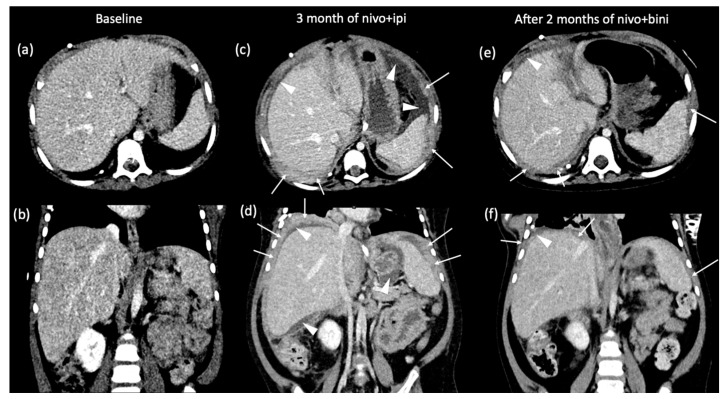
Axial (a) and coronal (b) contrast-enhanced CT of the abdomen at baseline are normal except for hepatomegaly. Axial (c) and coronal (d) contrast-enhanced CT of the abdomen after 3 cycles of nivolumab/ipilimumab demonstrating enhancing soft tissue along the peritoneum (arrows), compatible with peritoneal carcinomatosis, as well as moderate amount of ascites (arrowheads). Axial (e) and coronal (f) contrast-enhanced CT of the abdomen demonstrate significant improvement in ascites (arrowheads) and carcinomatosis (arrows) after 2 cycles of nivolumab/binimetinib.
